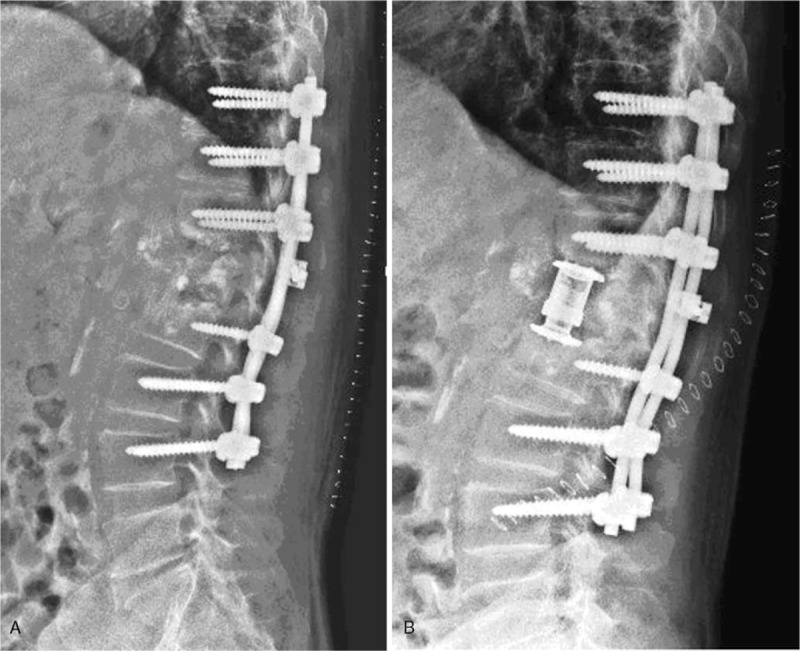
Lateral X-rays showing 2 steps of posterior pedicle internal fixation and anterior artificial vertebral body implantation immediately after reconstruction.
